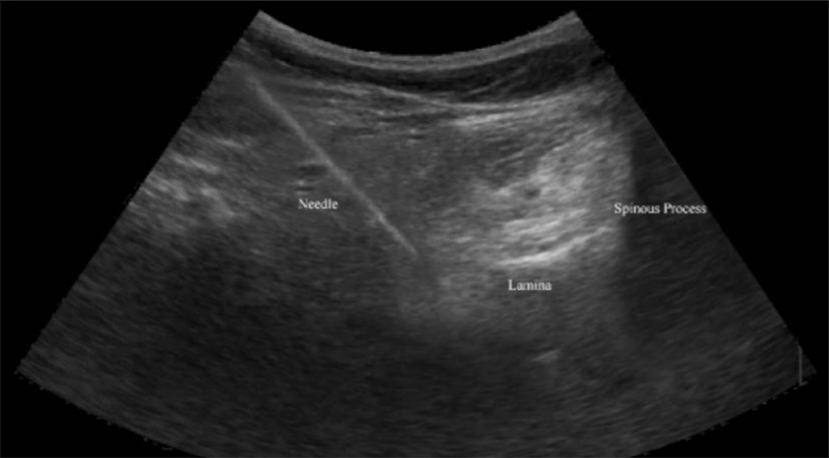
Ultrasound view of needle placement at L4/L5.
